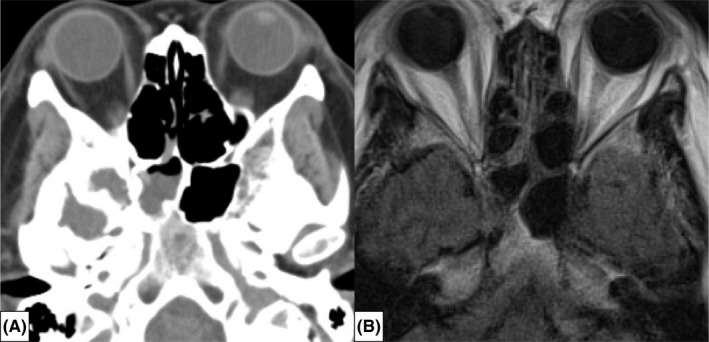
Brain images at the first examination. (A) Brain computed tomography (CT) with 4‐mm slice thickness showing fluid accumulation with air inclusion in the right sphenoid sinus. No fistula is detected. (B) Fluid‐attenuated inversion recovery magnetic resonance image (MRI) showing no evidence of deviation of the cranial contents into the sphenoid sinus
